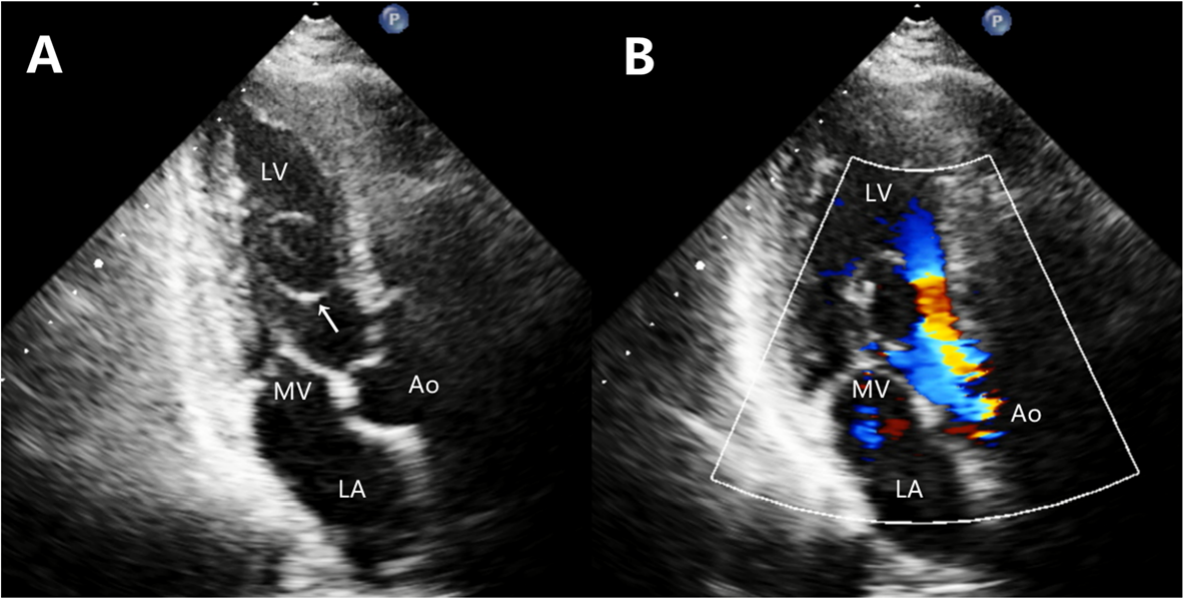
The preoperative echocardiography demonstrated (A) a highly mobile, hyperechogenic lesion (arrow) attached to the anterior mitral leaflet of the mitral valve, (B) the accelerated blood flow of the left ventricular outflow tract with mitral regurgitation. LV, left ventricle; Ao, aorta; MV, mitral valve; LA, left atrium
